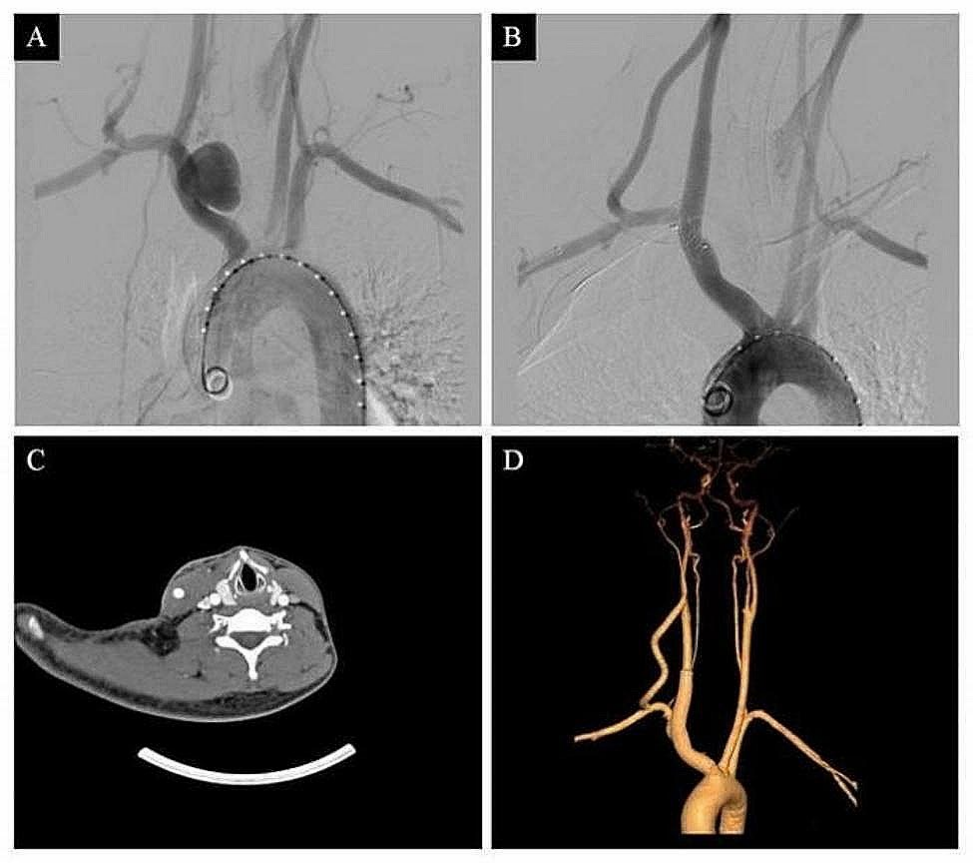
Representative images of (A) Intraoperative aortic arch angiography confirming the presence of a saccular aneurysm of the innominate artery involving the right proximal subclavian and common carotid arteries. (B) A combined endovascular and open-repair technique was used to cover the aneurysm, thereby avoiding the bleeding or endoleak. (C) Postoperative transverse computed tomography slide showing patency of both the right vertebral and graft arteries. (D) Postoperative follow-up CTA three-dimensional reconstruction of the aortic arch showing patency of the stent and right vertebral artery without endoleak or displacement
